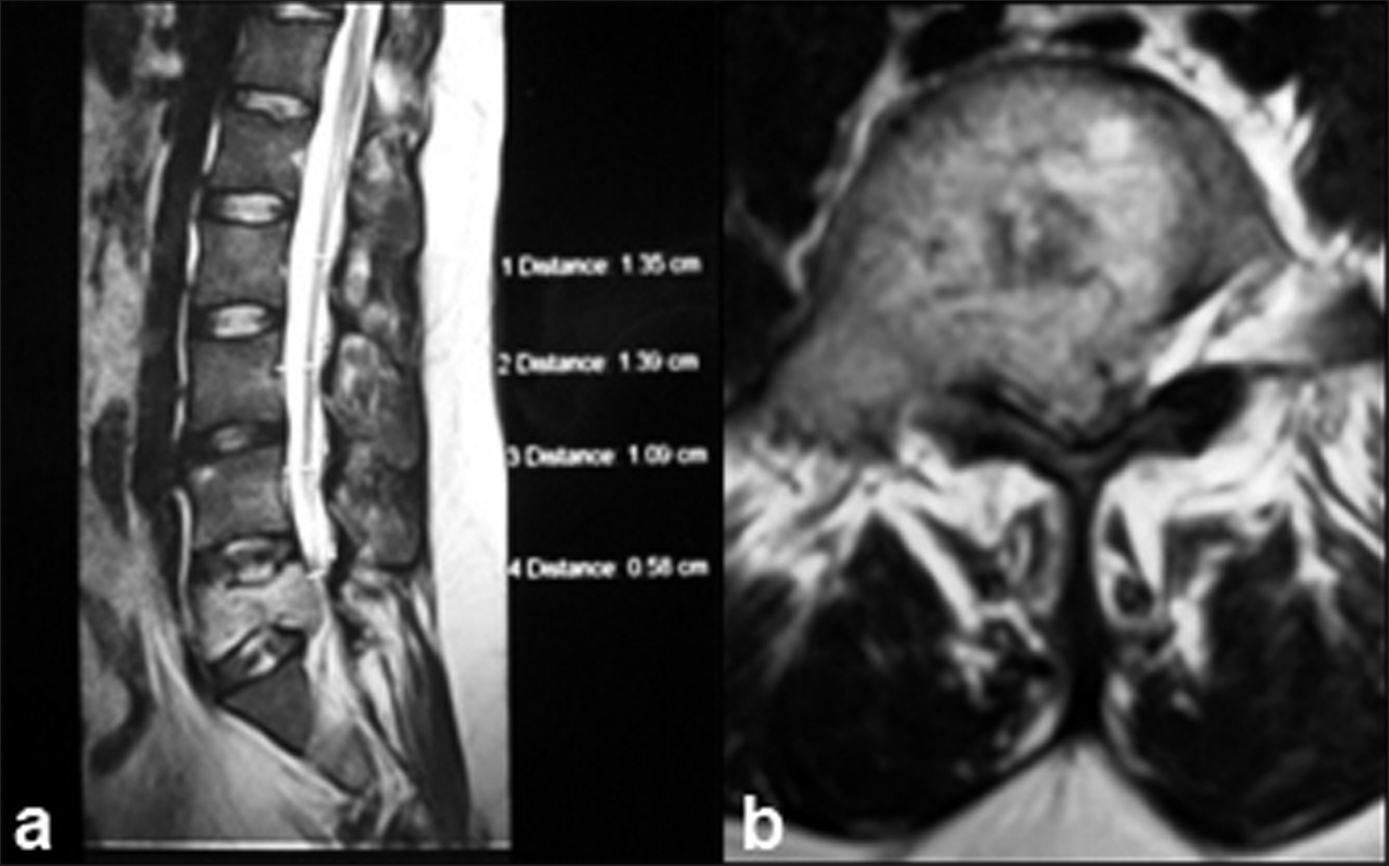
T_2_W MRI (a) saggital section showing cauda equina compression (b) Transverse section at L5 vertebral level showing complete involvement of the body, pedicles and extra spinal region
